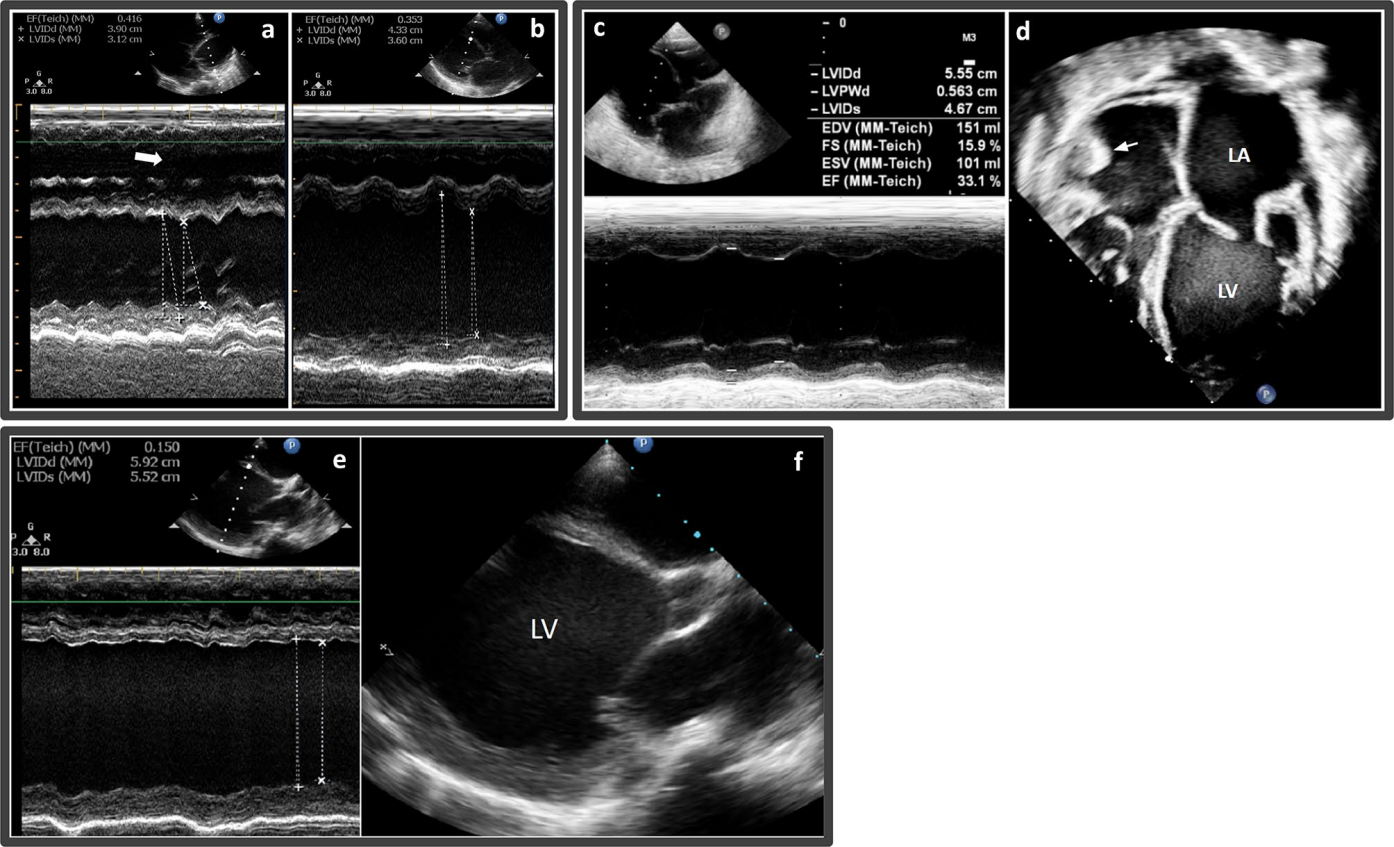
Echocardiographic images of patients with DCM. (a,b) M-mode echocardiographic images for 5-II-4 and 5-II-5 in Family F05. (a) 5-II-4, severe left ventricle dilatation with systolic dysfunction, with ejection fraction of 41%. The right ventricle is also dilated (white arrow) due to the presence of secundum ASD. (b) 5-II-5, severe left ventricle dilatation with systolic dysfunction, with an ejection fraction of 35%. c-d M-mode (c) and apical four-chamber (d) echocardiographic image of proband F06, showing severely dilated left ventricle (LV) and left atrium (LA), and depressed ventricular systolic function with ejection fraction (EF) of 33.1%. A right atrial thrombus is also seen (arrow). (e,f) Proband of Family F07. (e) M-mode and (f) longitudinal echocardiographic image of the left ventricle (LV) of a 2-year-old girl with dilated cardiomyopathy, showing severely dilated left ventricle, and severely depressed ventricular systolic function with ejection fraction (EF) of 15%.
